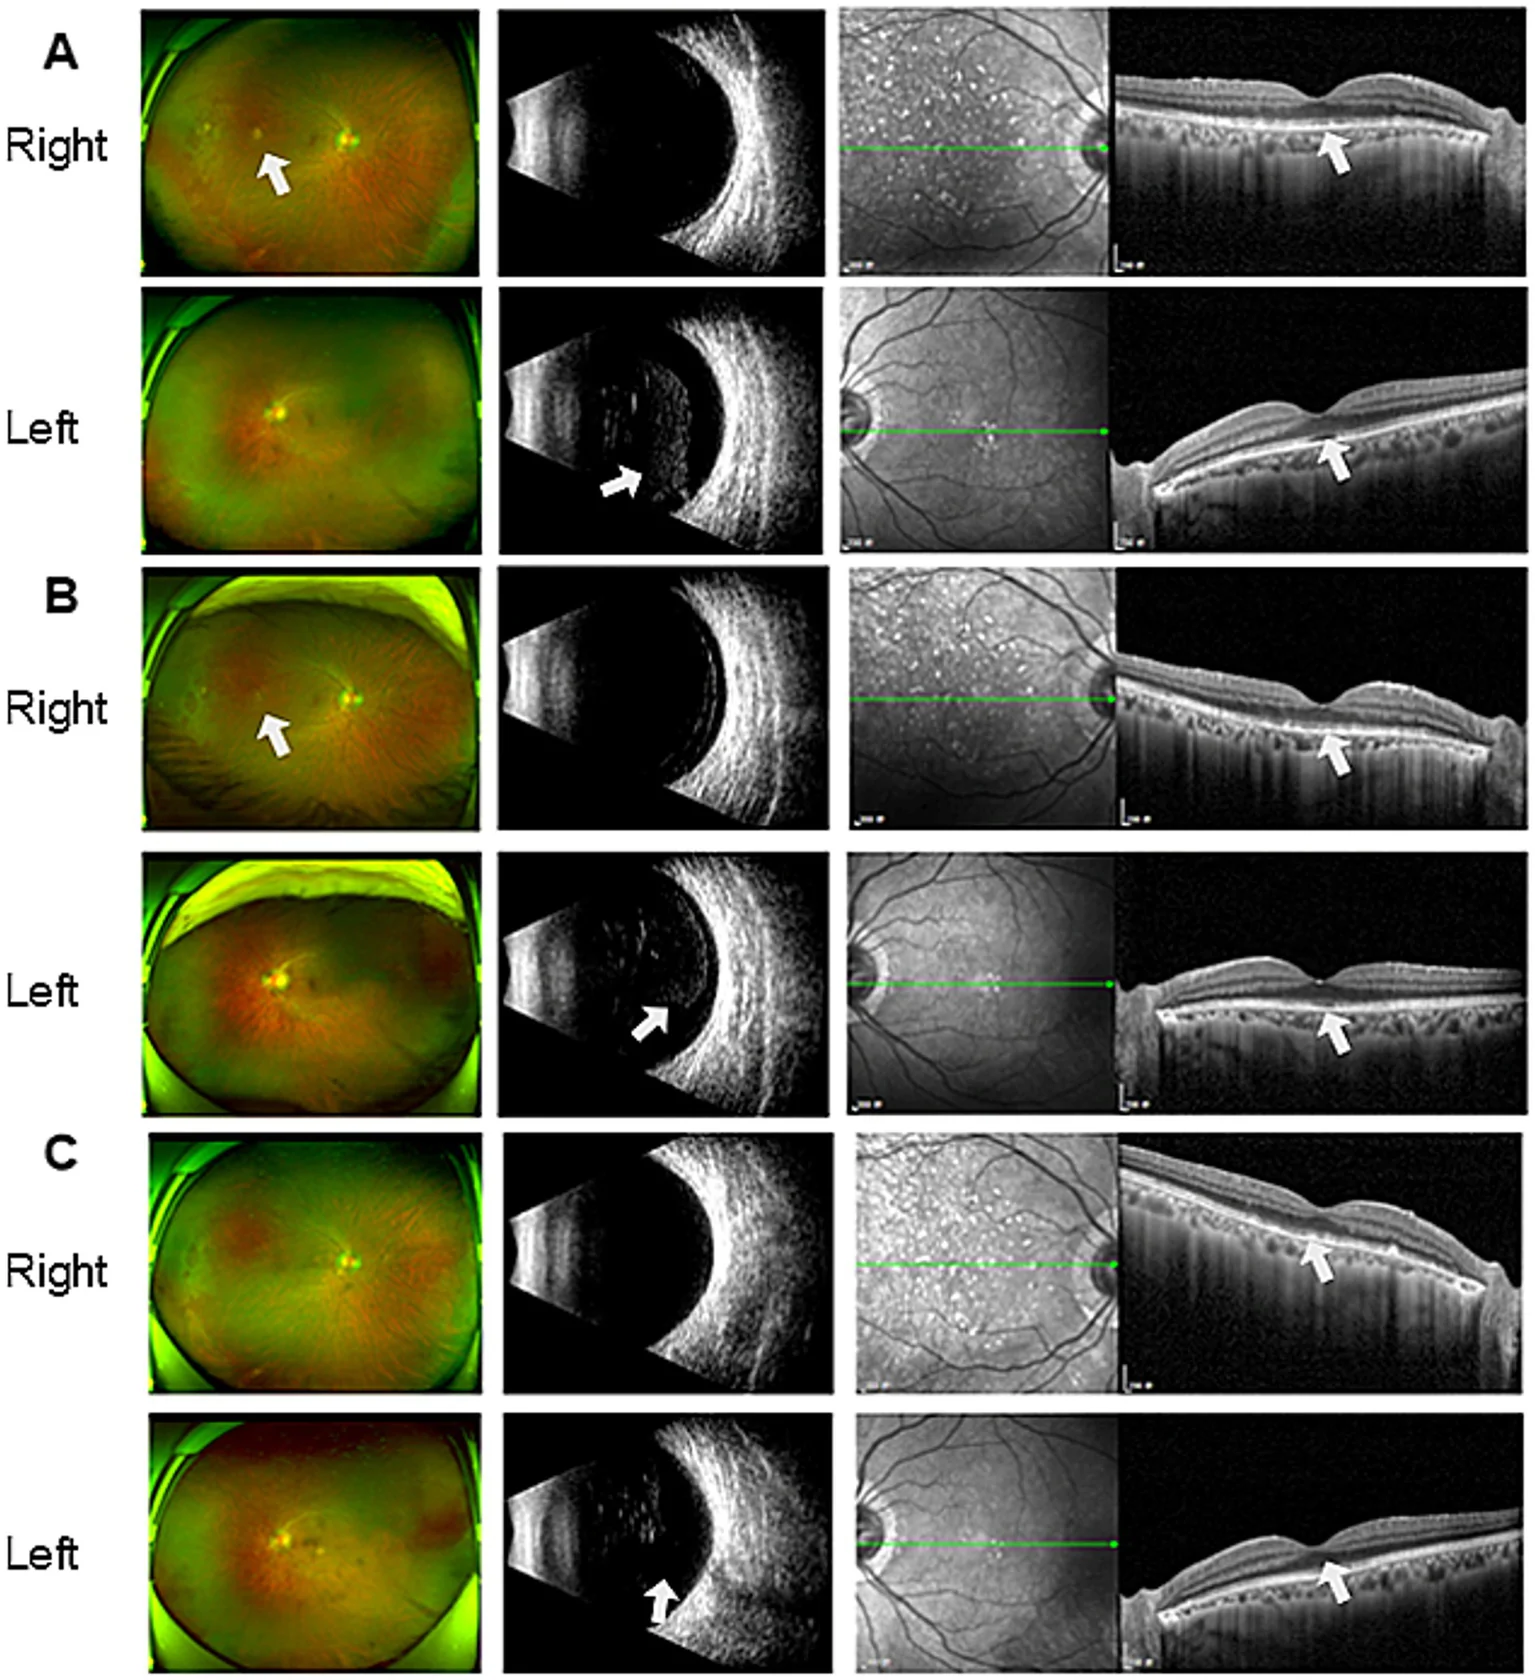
Fundoscopy, B-scan ultrasonography and optical coherence tomography (OCT) examinations during follow-up. (A) Results after oral medications treatment for 1 month on November 28th, 2022, the retinal infiltrate was significantly reduced compared to the onset of the disease, the vitreous opacity was relieved, and the structure of the outer layer of the retina has recovered better than before. (B) Results after oral medications treatment for 2 months on January 4th, 2023. The retinal infiltrate gradually disappeared, the vitreous opacity was relieved, the structure of the outer layer of the retina recovered. (C) Results after oral medications treatment for 6 months on May 10th, 2023, the retinal infiltrate completely disappeared, and the vitreous opacity was significantly relieved, the structure of the outer layer of the retina has mostly recovered, especially in the left eyes.
